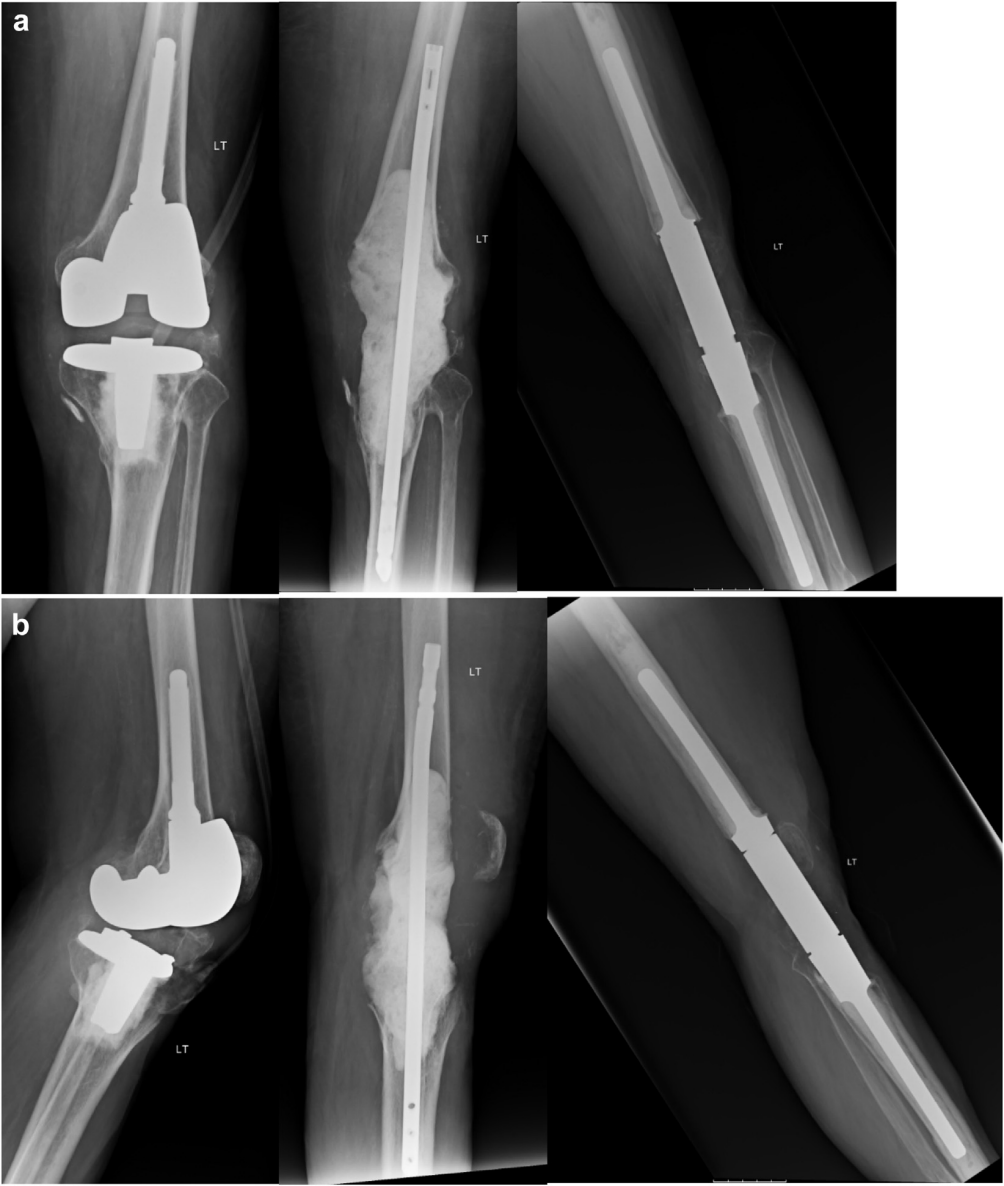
Radiographs of a representative case. Anteroposterior (a) and lateral (b) views depicting presentation, static antibiotic spacer placement, and final construct.
